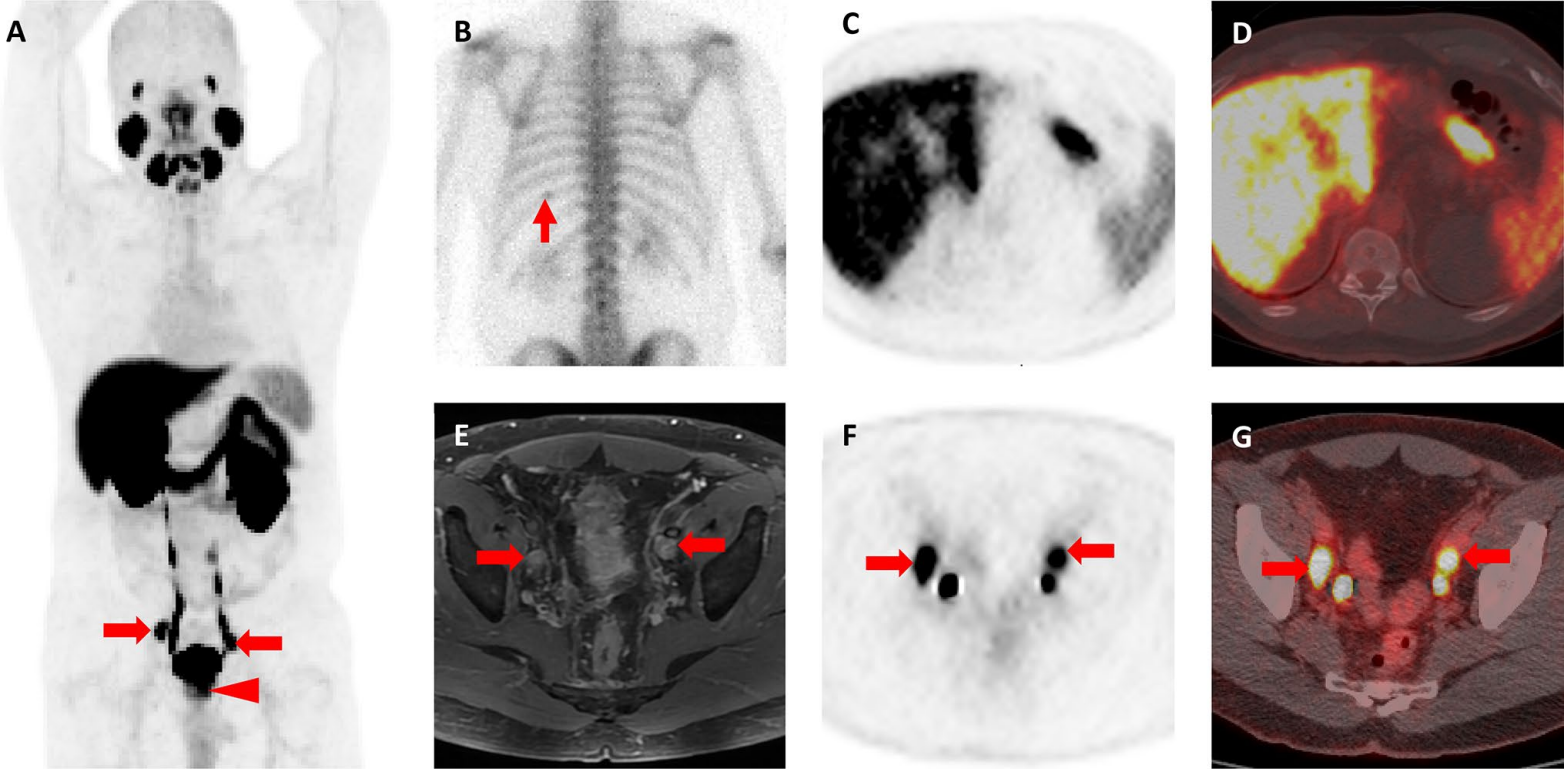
a Whole-body maximum intensity projection ^18^F-DCFPyL PET image demonstrates intense uptake in the patient’s primary tumor (red arrowhead) and bilateral pelvic lymph nodes (red arrows). No abnormal uptake is appreciated in the chest. b Posterior planar ^99m^Tc-methylene diphosphonate bone scan image shows abnormal uptake in the posterior left tenth rib (thin red arrow). This was considered suspicious in light of the known high-risk diagnosis. c Axial ^18^F-DCFPyL PET and d^18^F-DCFPyL PET/CT images show no evidence of abnormal uptake in the posterior left tenth rib. e Axial T1-weighted, post-contrast MRI of the pelvis demonstrates bilateral enlarged and enhancing pelvic lymph nodes (red arrows). f Axial ^18^F-DCFPyL PET and g^18^F-DCFPyL PET/CT show intense uptake in the bilateral pelvic lymph nodes (red arrows). The additional foci of uptake in (f) and (g) represent excreted radioactive urine in the distal ureters
